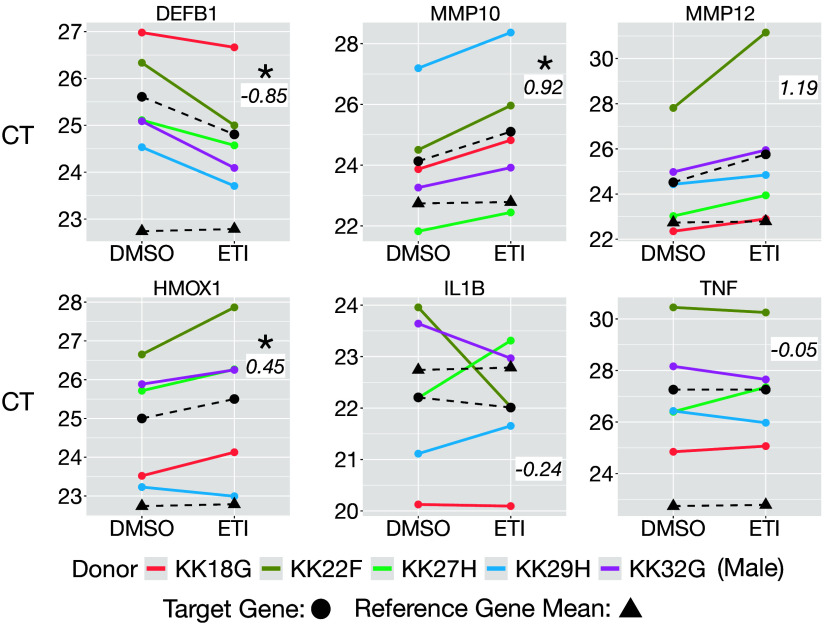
qPCR measurements of *DEFB1, MMP10, MMP12, HMOX1, IL1B, TNF,* and reference genes. Cycle threshold (CT) mean values of each target gene (black circles connected by a dashed line) and reference gene mean values (black triangles connected by a dashed line) are presented. CT values of the gene of interest are shown for each donor (colors). The figure shows that the average of the three reference genes (*HSP90AB, GAPDH,* and *HPRT1*) was neither induced nor repressed in response to ETI: the slope of dashed lines connecting the mean of reference genes in the two conditions is close to zero. *DEFB1, MMP10, MMP12,* and *HMOX1* were significantly differentially expressed in response to ETI using a mixed-effect linear model of the ETI effect on each target gene accounting for the mean of the reference genes with donor modeled as a random effect. *DEFB1*: *P* = 0.02, *MMP10*: *P* = 0.03, *MMP12*: *P* = 0.09, *HMOX1*: *P* = 0.02, IL1B: *P* = 0.79, TNF: *P* = 0.99. Delta-delta CT values for each target shown in italics in each panel. P* < 0.05. A positive ΔΔCT value of 1 corresponds to a twofold (200%) increase in abundance in the gene of interest. Donor KK32G (purple) is male. ETI, elexacaftor/tezacaftor/ivacaftor.
